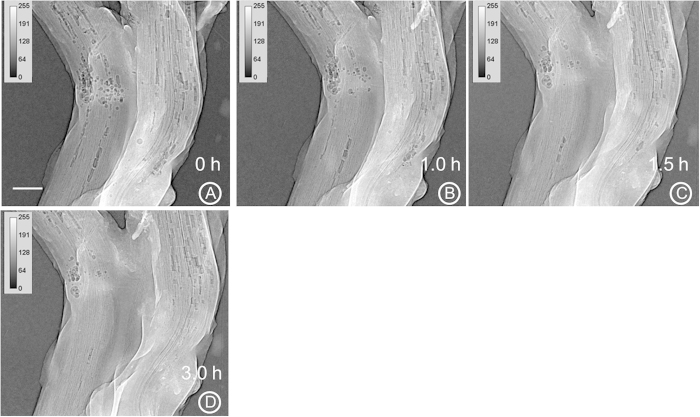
Use of PCI to determine the rate of water movement through a canola stem. X-ray image recorded before introduction of water (A) and series of images (B–D) recorded at different times after introduction of iodine and water. X-ray energy used was 18 keV, exposure time was 1 s and effective pixel size was 8.75 μm. Scale bar = 0.7 mm.
